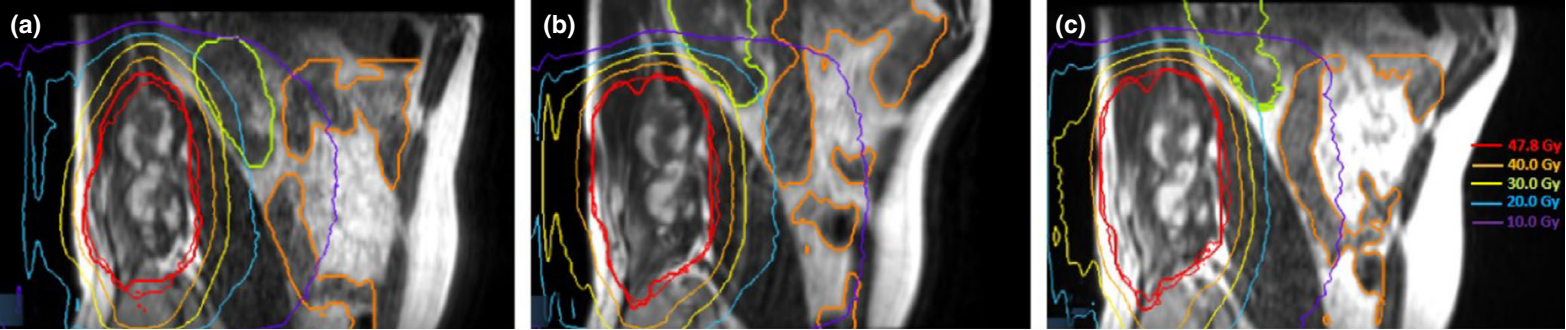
Sagittal views of planning magnetic resonance imaging (MRI) MRIdian true fast imaging with steady state precession sequences with dose distribution in deep inspiration breath hold (a), free breathing (b), and final expiration breath hold (c) conditions.
